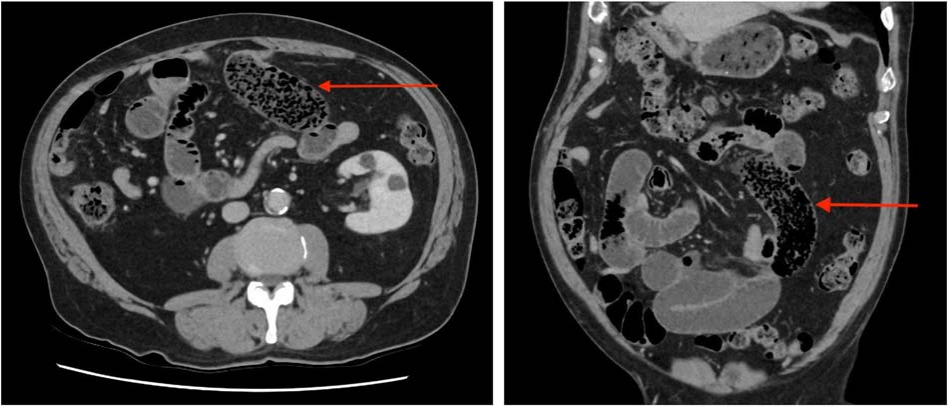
Abdominal CT scan axial (left) and coronal (right) images demonstrating ‘faecalization’ (arrow) of small bowel content.
